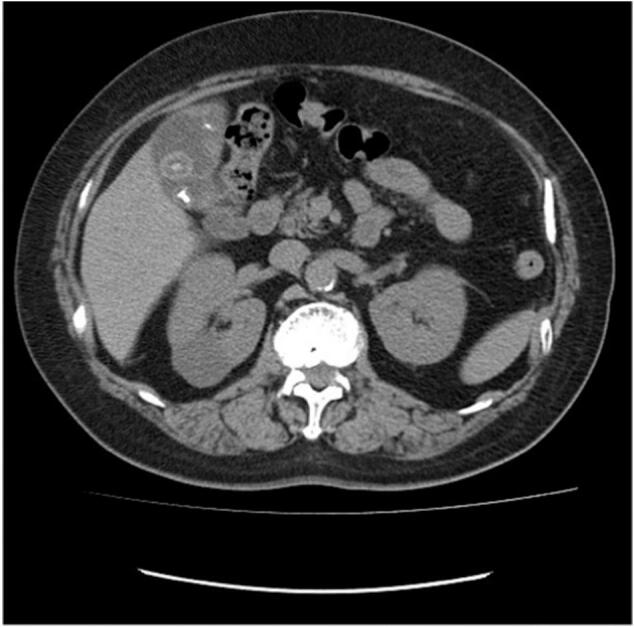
Computed tomography imaging taken 7 months before surgery.
